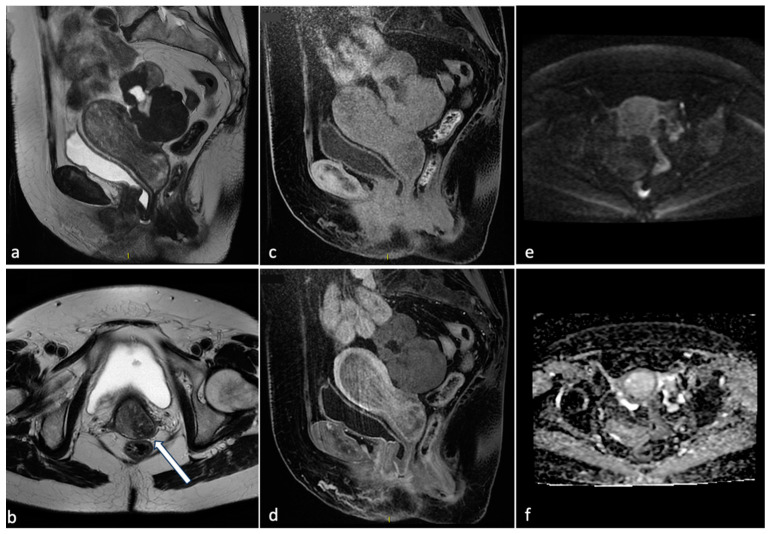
Voluminous endometrial polyp. A 76-year-old woman with post-menopausal bleeding. MRI shows on T2 sequences (a,b) a voluminous mass within the endometrial cavity of intermediate signal intensity with cystic components and a fibrous core (arrow). The mass demonstrates absence of endometrial invasion on pre- and post-contrast T1 sequences (c,d) and low signal on DWI with high signal on the ADC map (e,f). These findings are suggestive of partially expelled endometrial polyp.
